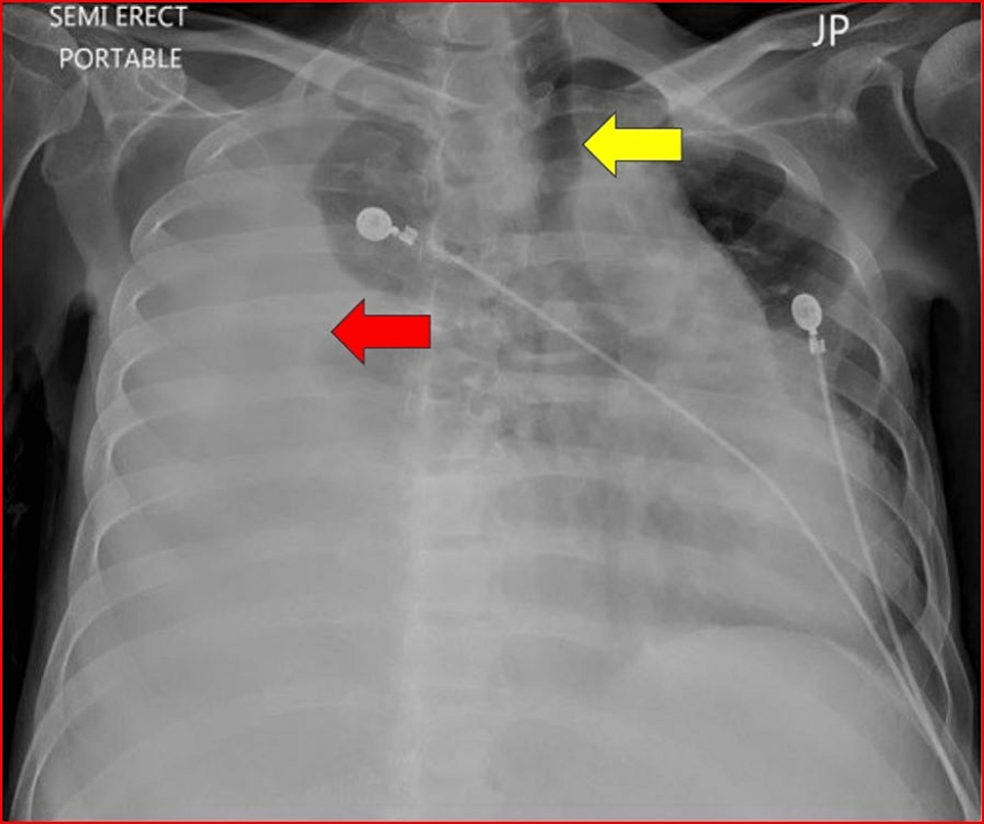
Chest X-ray with right-sided homogenous opacification (red arrow) and mediastinal shift to the opposite side (yellow arrow) suggesting an effusion.
